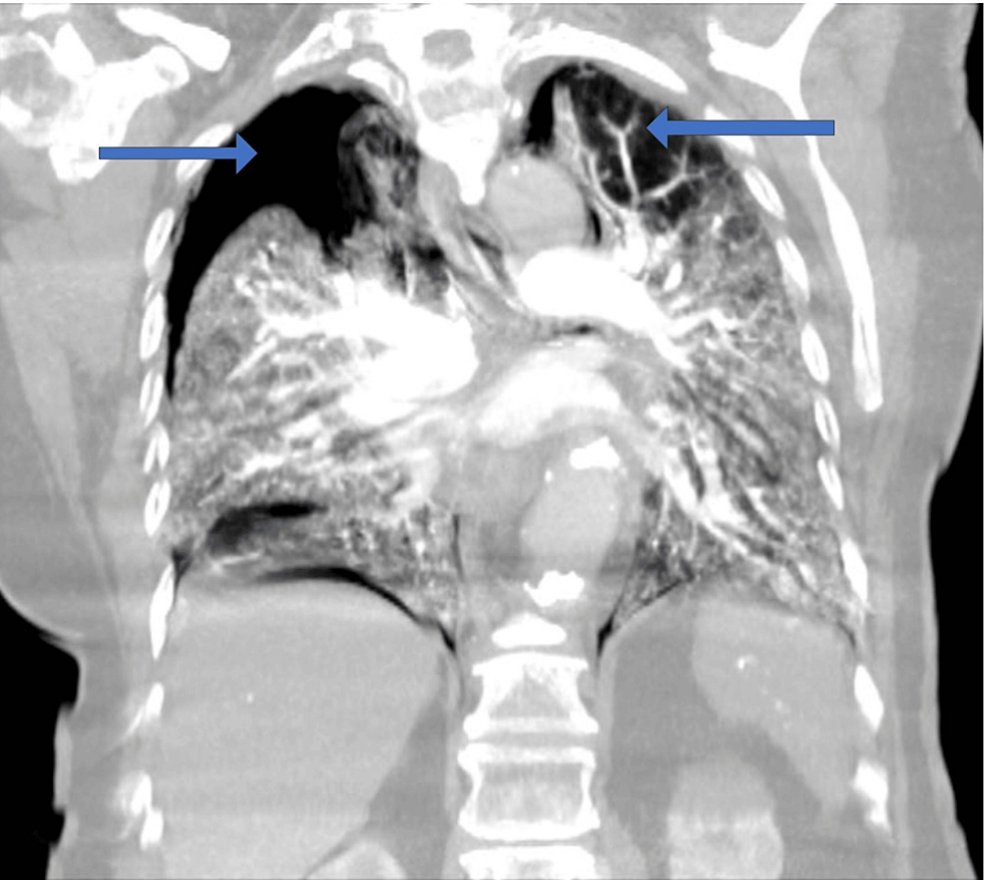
Repeat CT angiography of the chest showing bilateral pneumothorax with right greater than left.
